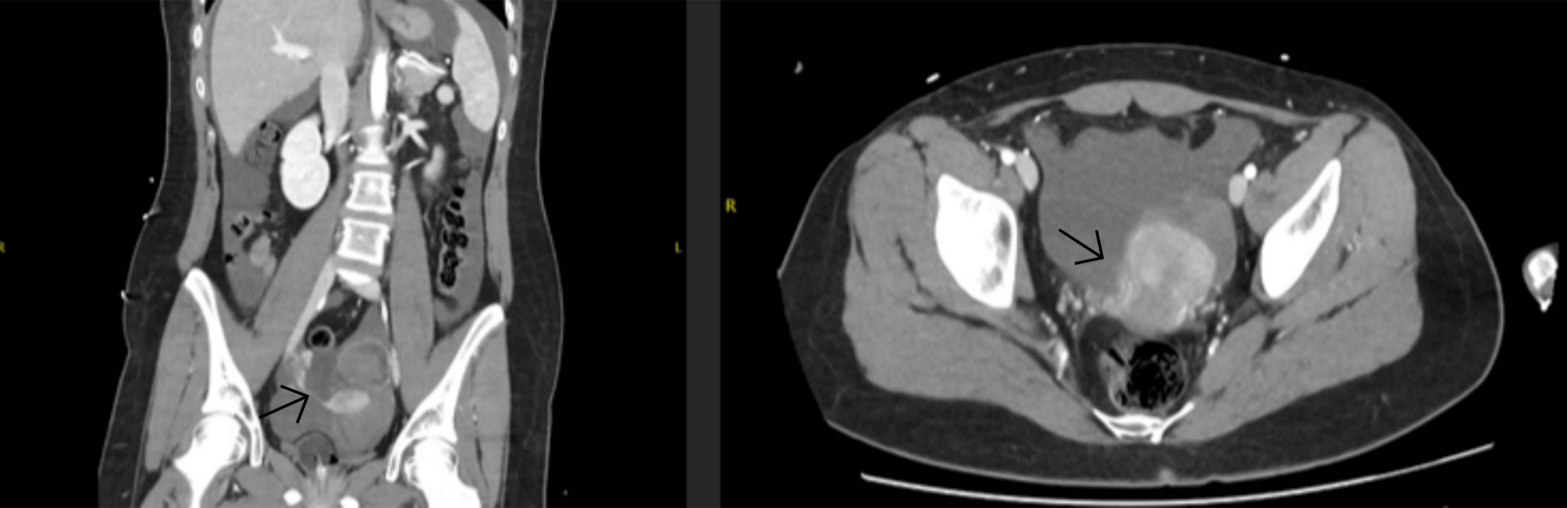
A CT of the abdomen and pelvis showing bleeding from the left ovary/ adnexa
